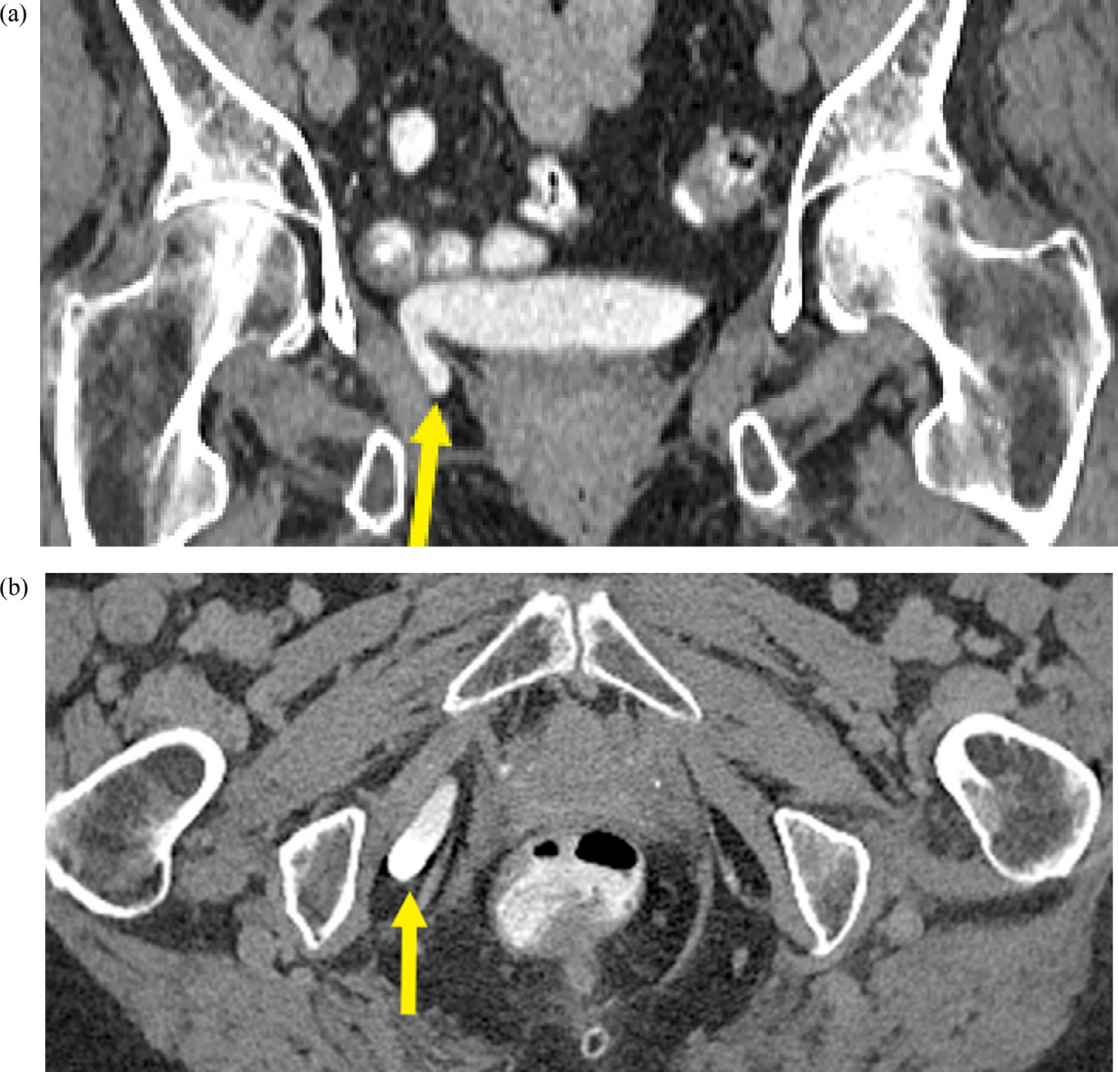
(a) Coronal and (b) axial CT images in the delayed phase demonstrate a right-sided bladder hernia containing contrast material (yellow arrow) extending through the pudendal canal (color version of figure is available online).
